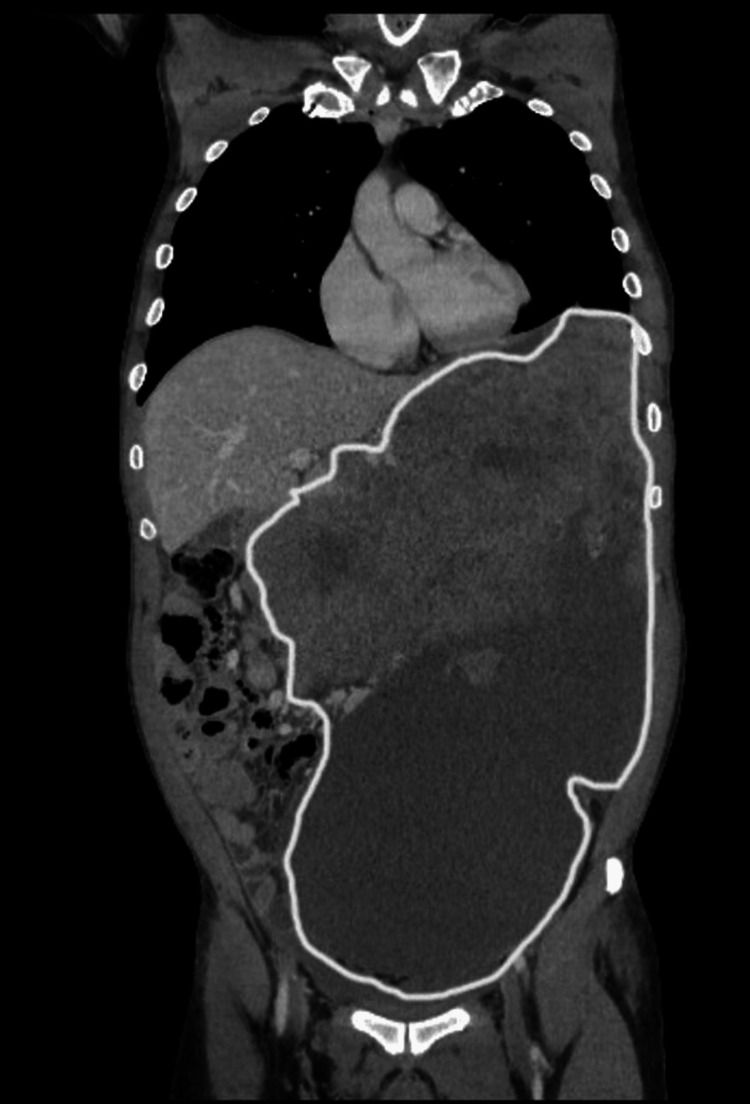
Abdominal CT images before neoadjuvant therapy with imatinib show an intraabdominal mass occupying most of the abdominal pelvic cavity, causing a significant mass effect. The mass shifts the bowel loops to the right side of the abdomen, compresses the retroperitoneal structures, and pushes the stomach inferiorly. It measures 35 x 25 x 20 cm and originates from the fundus of the stomach.
